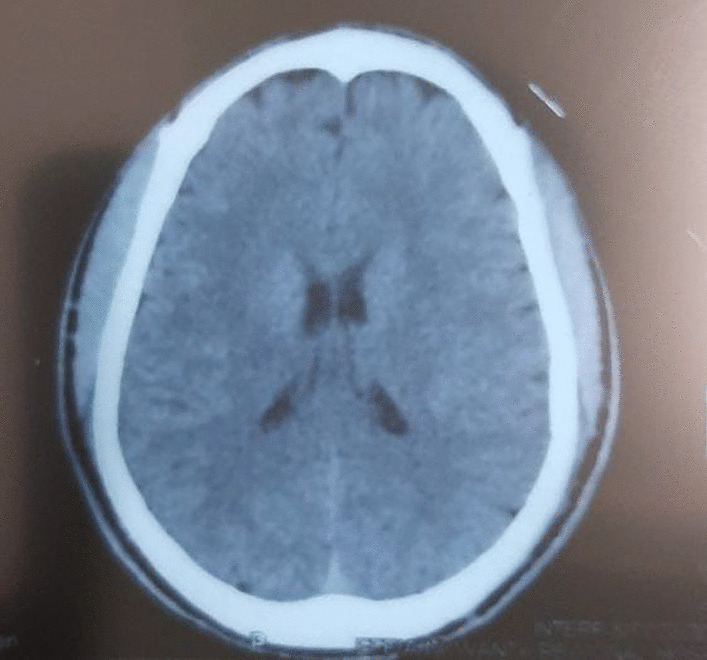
Head Computed Tomography scan of patient
